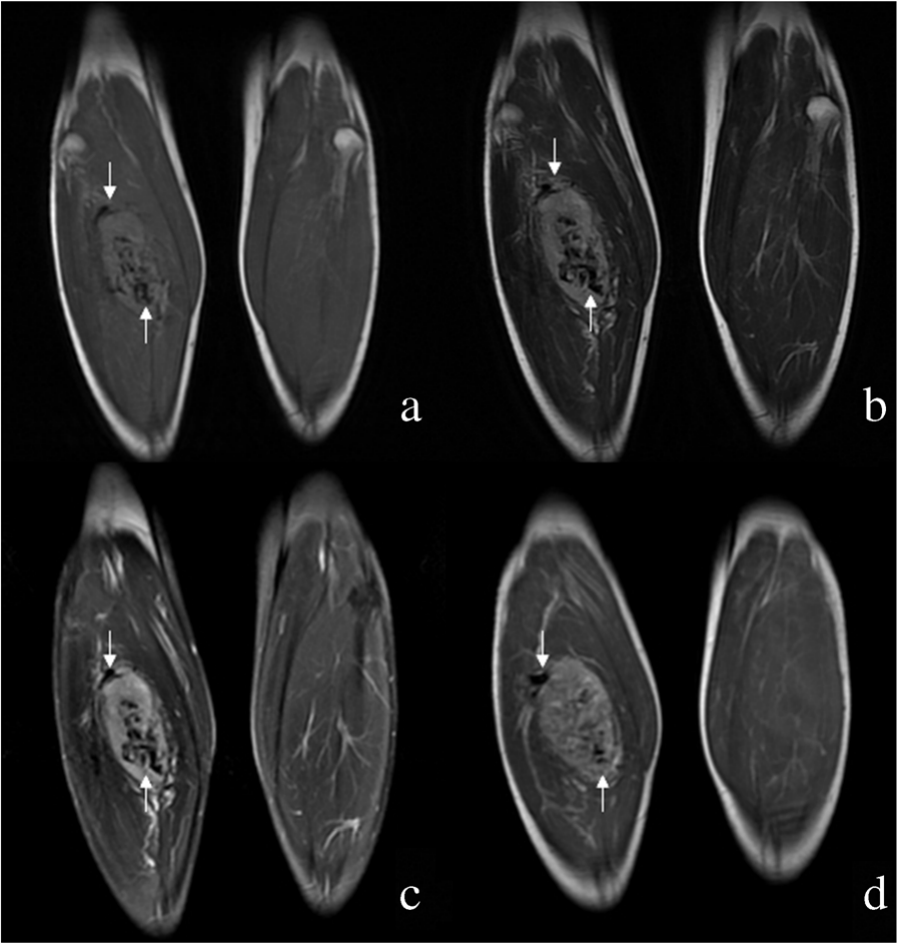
A 25-year-old man with alveolar soft part sarcoma. (a) Coronal T1-weighted image shows a hyperintense signal tumor in the right lower calf with an ill-defined margin in a 25-year-old man. There are tortuous intra- and extratumoral signal voids (arrows). (b) Coronal T2-weighted image and (c) coronal fat-suppressed T2-weighted image reveal the hyperintense signal tumor with intra- and extratumoral signal voids (arrows). (d) Coronal post-contrast T1-weighted image displays inhomogeneous enhancement of the tumor with tortuous vessel (arrows).
